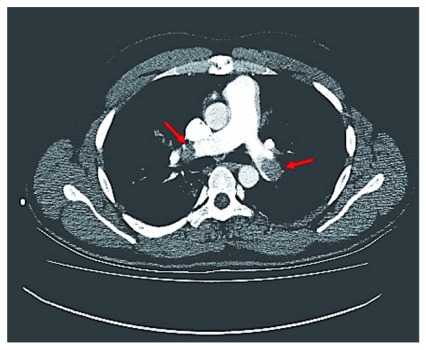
CT Pulmonary Angiogram showing non-lumen occluding thrombus (indicated by red arrows) in the right and left main pulmonary trunk.
